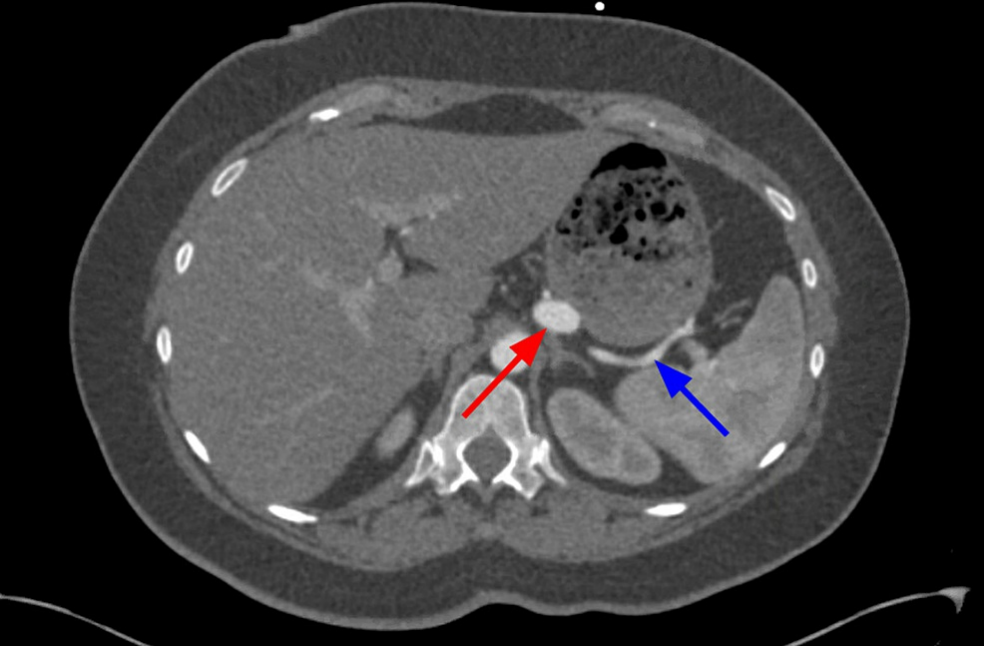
Axial view of CTA abdomen and pelvis demonstrating the splenic artery (blue arrow) with an aneurysm in the proximal part of the splenic artery (red arrow). CTA, computed tomography angiography
